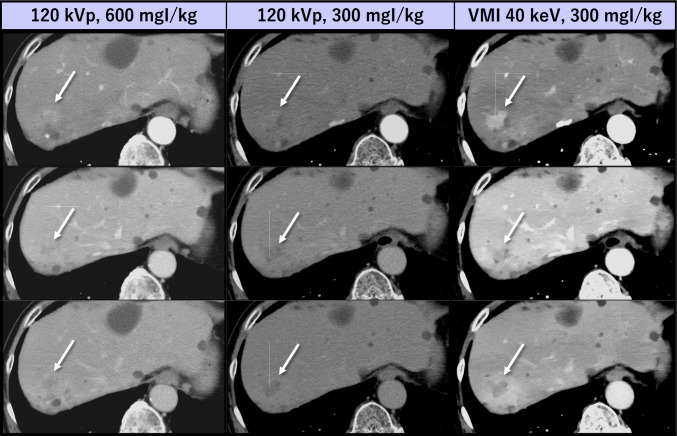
A 78-year-old man with hepatocellular carcinoma underwent multiphase hepatic CT with both standard (600 mgI/kg) and low (300 mgI/kg) ICM dose protocols on single-energy and dual-layer detector CT scanners, respectively, at 6 months interval. Compared with the standard protocol, better lesion conspicuity was attained at 40 keV VMI even with a 50% reduction in ICM dose without increasing radiation dose (size specific dose estimate: 15.4 vs. 14.7 mGy)
